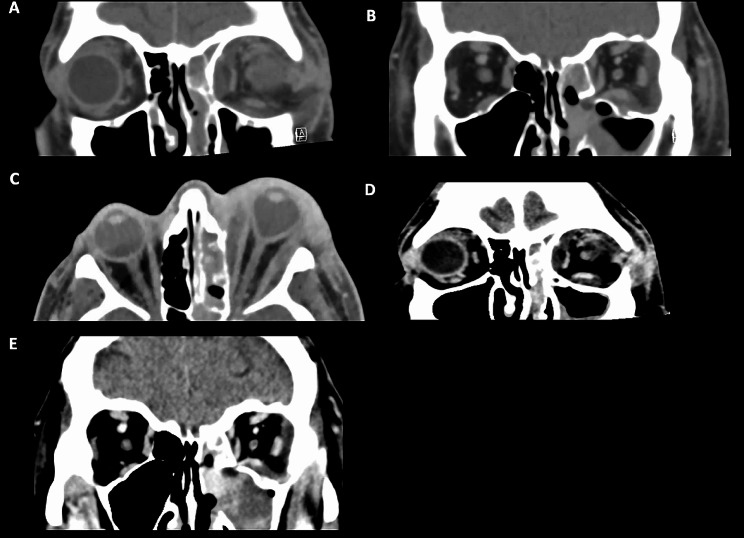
Case 3: A-B: Coronal CT image demonstrating subperiosteal collection along the left medial wall, orbital floor and lateral wall. C: Axial CT image demonstrating significant tenting of the left globe. D-E: A repeat CT orbital scan revealed persistent circumferential subperiosteal collections of the left orbit
